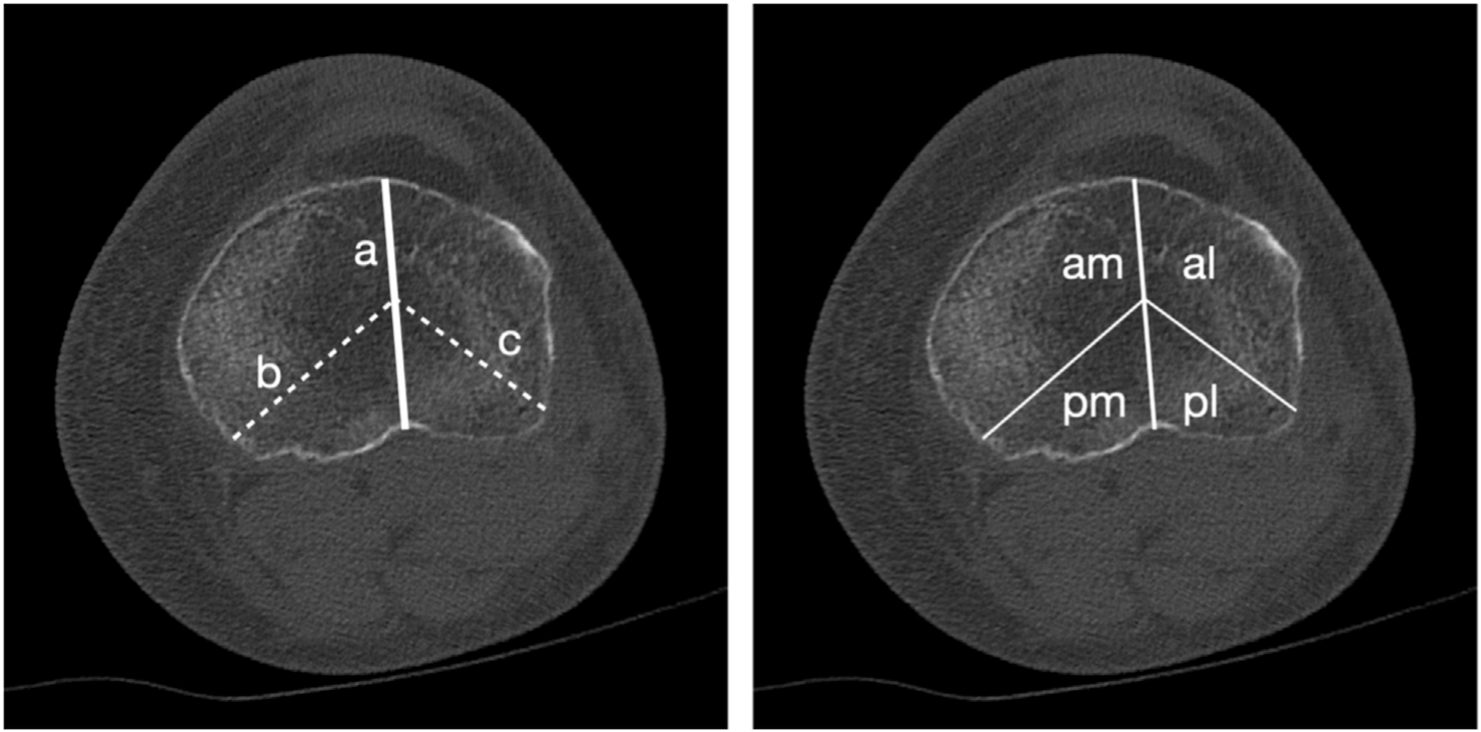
Axial slice of a CT scan of the tibial plateau, just above the tip of the fibula. Left side shows three lines: a, from the tibial tubercle to the posterior cruciate sulcus; b, from the posteromedial cortex to the midpoint of line a; and c, from the region of the fibula in the posterolateral corner to the midpoint of line a. Right side shows the same slice, now marking.
